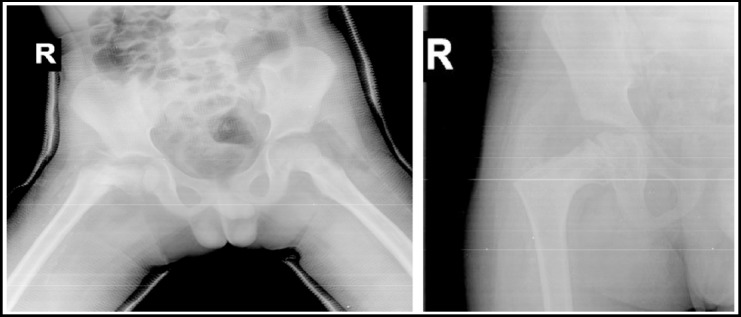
X-rays after spica application and 3 months post op.
